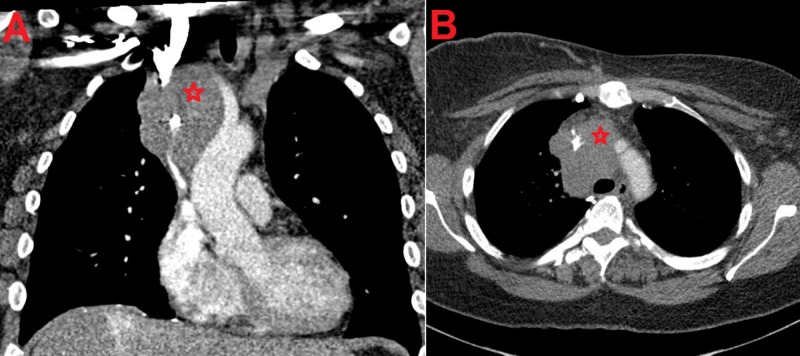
Computed tomography of the chest with intravenous contrast at presentation Coronal (A) and axial (B) section of the chest showing the circumferential compression of the superior vena cava by the anterior mediastinal mass (red star)
